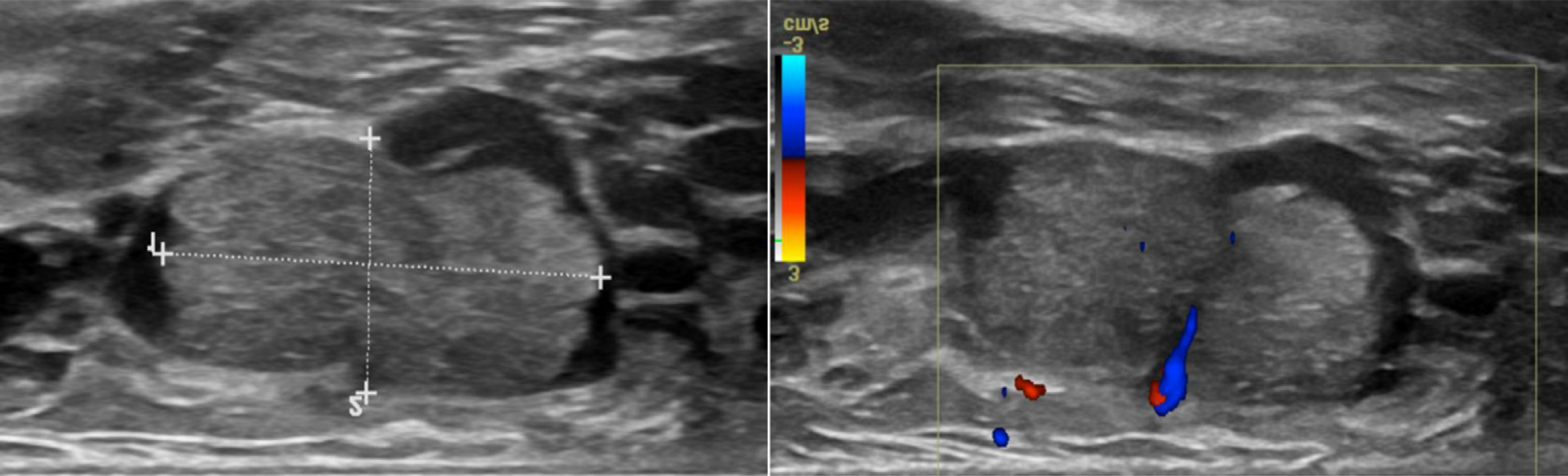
Greyscale ultrasound examination shows intraductal homogeneous solid lesion at 9:00 peri areolar region measuring 2.3 × 1.7 × 1.2 cm. Note the dilated anechoic duct containing the lesion. Color Doppler shows internal vascularity within the lesion.
